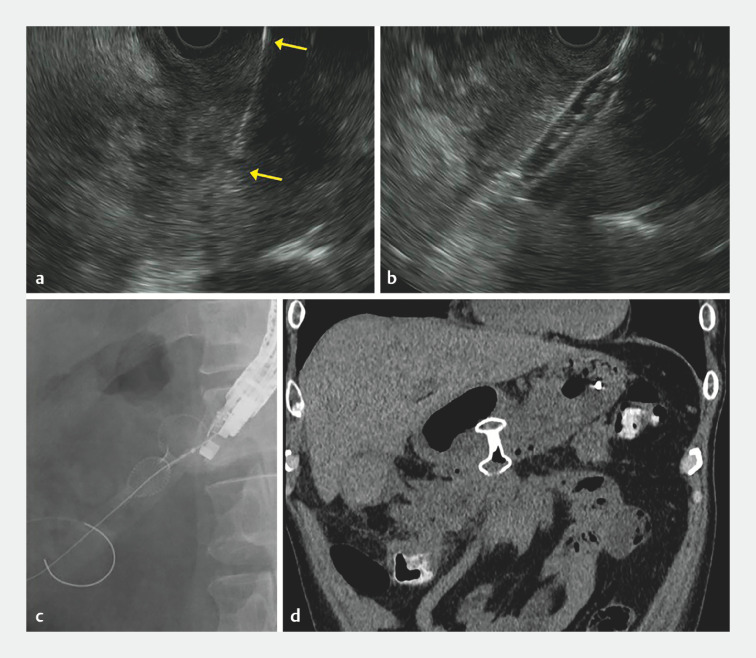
Endoscopic ultrasound-guided placement of a lumen-apposing metal stent (LAMS) for walled-off necrosis (WON) using the balloon traction technique.
